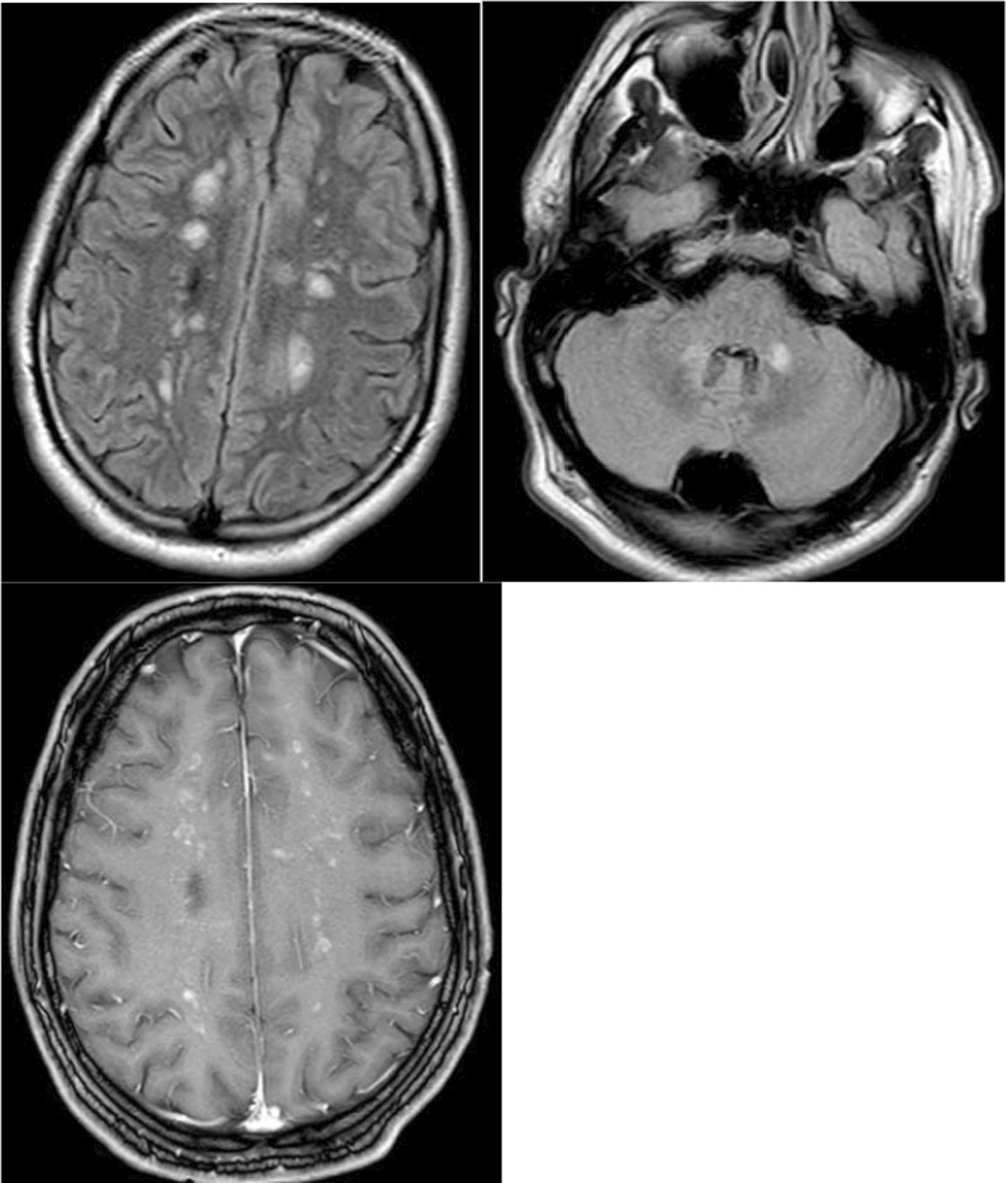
MRI at admission. The FLAIR sequence depicts multiple hyperintense infra- and supratentorial white matter lesions. Patchy contrast enhancement of all lesions is visible on contrast enhanced T1 weighted imaging
